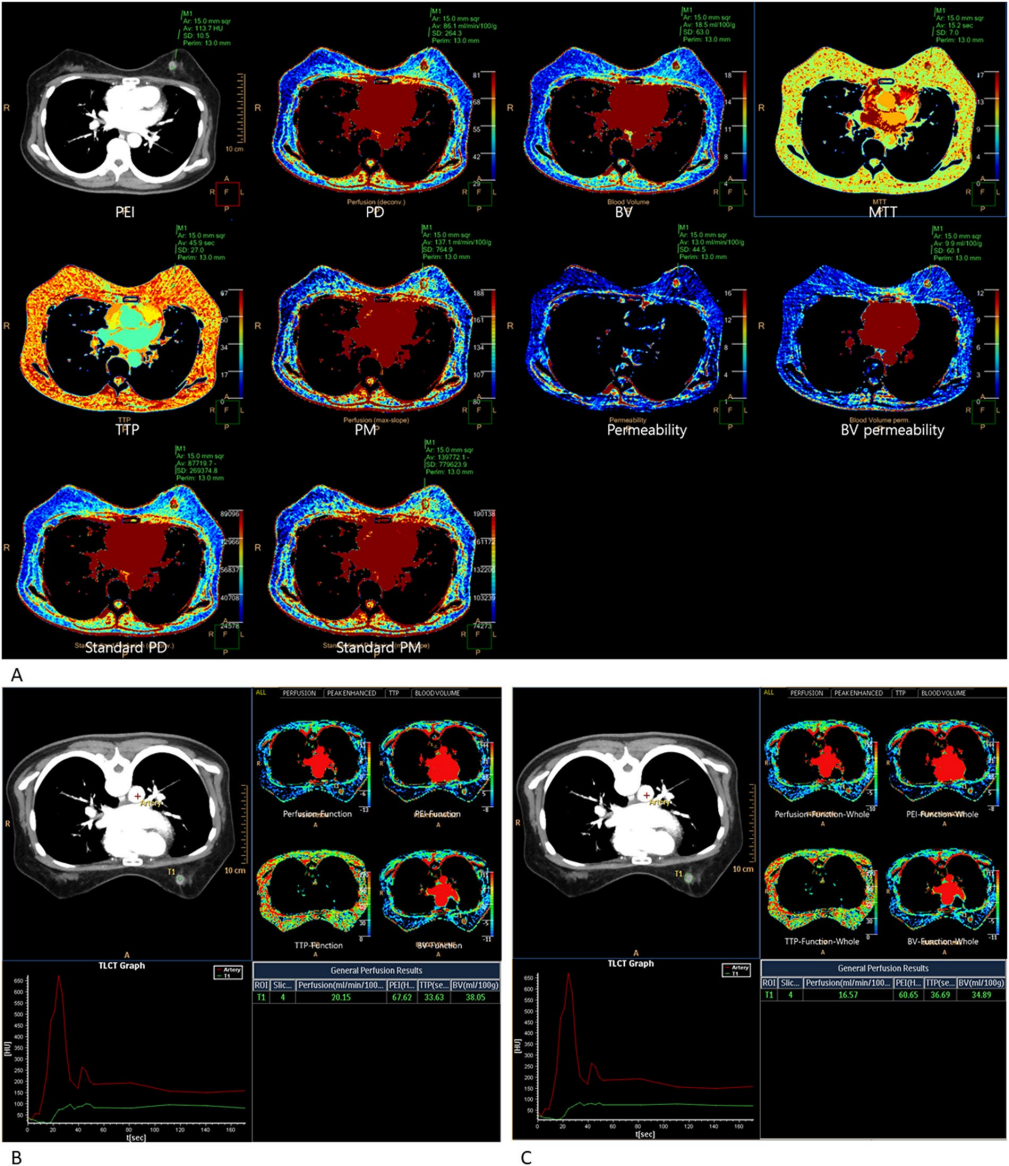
Evaluated 18 hemodynamic parameters on low-dose breast perfusion CT. (A) Ten parameters were obtained at the hot spot of the tumor using the Advanced Perfusion and Permeability Application software; peak enhancement intensity (PEI), perfusion on deconvolution model (PD), perfusion on maximum slope model (PM), blood volume (BV), mean transit time (MTT), time to peak (TTP), permeability (Permeability), blood volume permeability (BV permeability), standardization of perfusion on deconvolution model (Standard PD), and standardization of perfusion on maximum slope model (Standard PM). (B) Four parameters were obtained at the hot spot of the tumor using the Functional CT software; perfusion (Perfusion-Function), PEI (PEI-Function), TTP (TTP-Function), and BV (BV-Function). (C) Four parameters were obtained at the whole tumor range using the Functional CT software; perfusion of whole breast cancer (Perfusion-Function-Whole), PEI of whole breast cancer (PEI-Function-Whole), TTP of whole breast cancer (TTP-Function-Whole), and BV of whole breast cancer (BV-Function-Whole).
